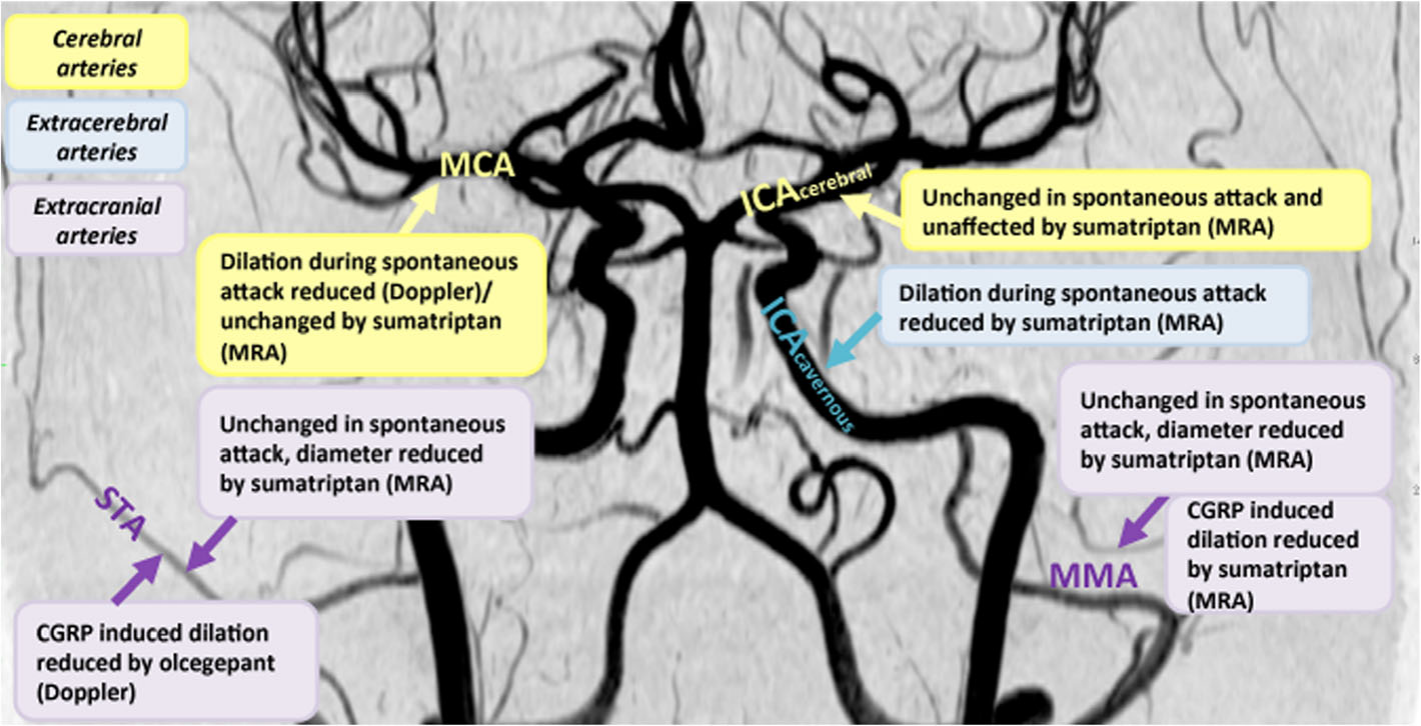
Effects of sumatriptan and olcegepant on cranial vessels in migraine patients. The intracerebral vessels, the middle cerebral artery (MCA) and the cerebral part of the internal carotid artery (ICA_cerebral_), are both shown in yellow. The extracerebral artery, the cavernous part of the internal carotid artery (ICA_cavernous_), is shown in blue. The extracranial vessels, the middle meningeal artery (MMA) and the superficial temporal artery (STA), are both shown in purple. Boxes include description of vessel reactivity during spontaneous and/or CGRP induced attacks as well as vessel response to sumatriptan and/or olcegepant. Imaging modality indicated in parentheses in boxes; magnetic resonance angiography (MRA) or transcranial ultrasound Doppler (Doppler). Image from MRA of healthy volunteer kindly provided by Faisal M Amin
